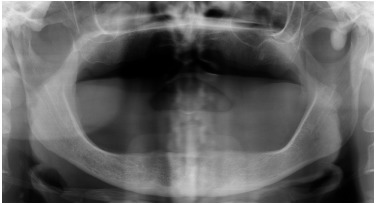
Panoramic radiographic visualizing a pedunculated osseous lesion originating from the left zygomatic arch.
